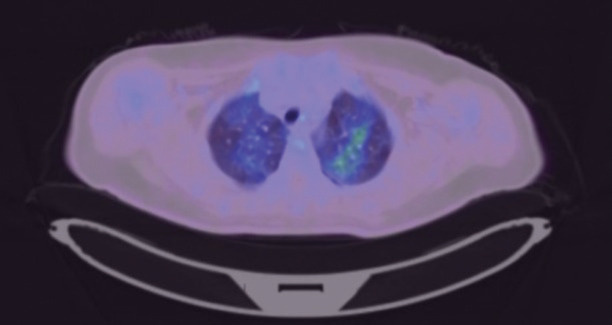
PET-CT showing FDG-avid diffuse GGOs in both lung fields.
